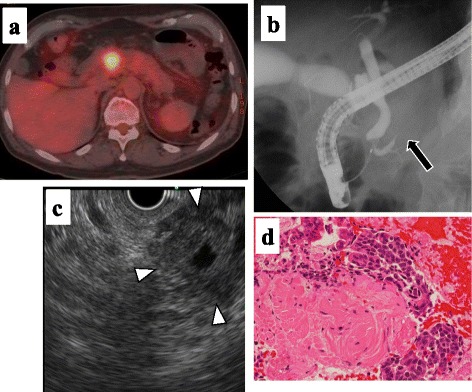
PET-CT revealed increased accumulation in the mass of the pancreas head (maximum standardized uptake value (SUV max): 5.6 (initial) and 7.8 (delayed) (a). Endoscopic retrograde cholangiopancreatography (ERCP) showed severe narrowing and obstruction of the main pancreatic duct by the tumor (*arrow*) (b). Endoscopic ultrasonography (EUS) showed the tumor as a low echoic mass (c). Cytological analysis by means of EUS-guided fine-needle aspiration (FNA) suggested a neuroendocrine tumor (G2) (d)
